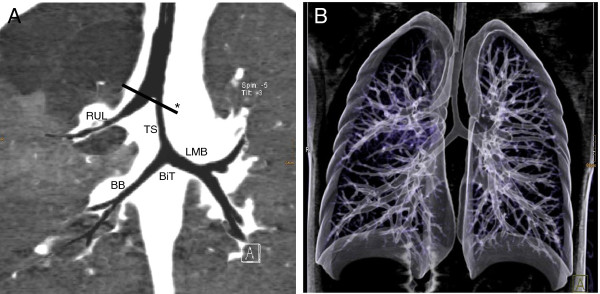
A) 2-dimensional and B) 3-dimensional computed tomography reconstruction of the tracheo-bronchial system. Bridging bronchus (BB) originating in the left main-stem bronchus (LMB) crossed the mediastinum and provided the right lower and middle lobe. Trachea (T), right upper lobe bronchus (RUL), bifurcatio tracheae (BiT), tracheal stenosis (TS). Appropriate view of Figure 1 (*).
